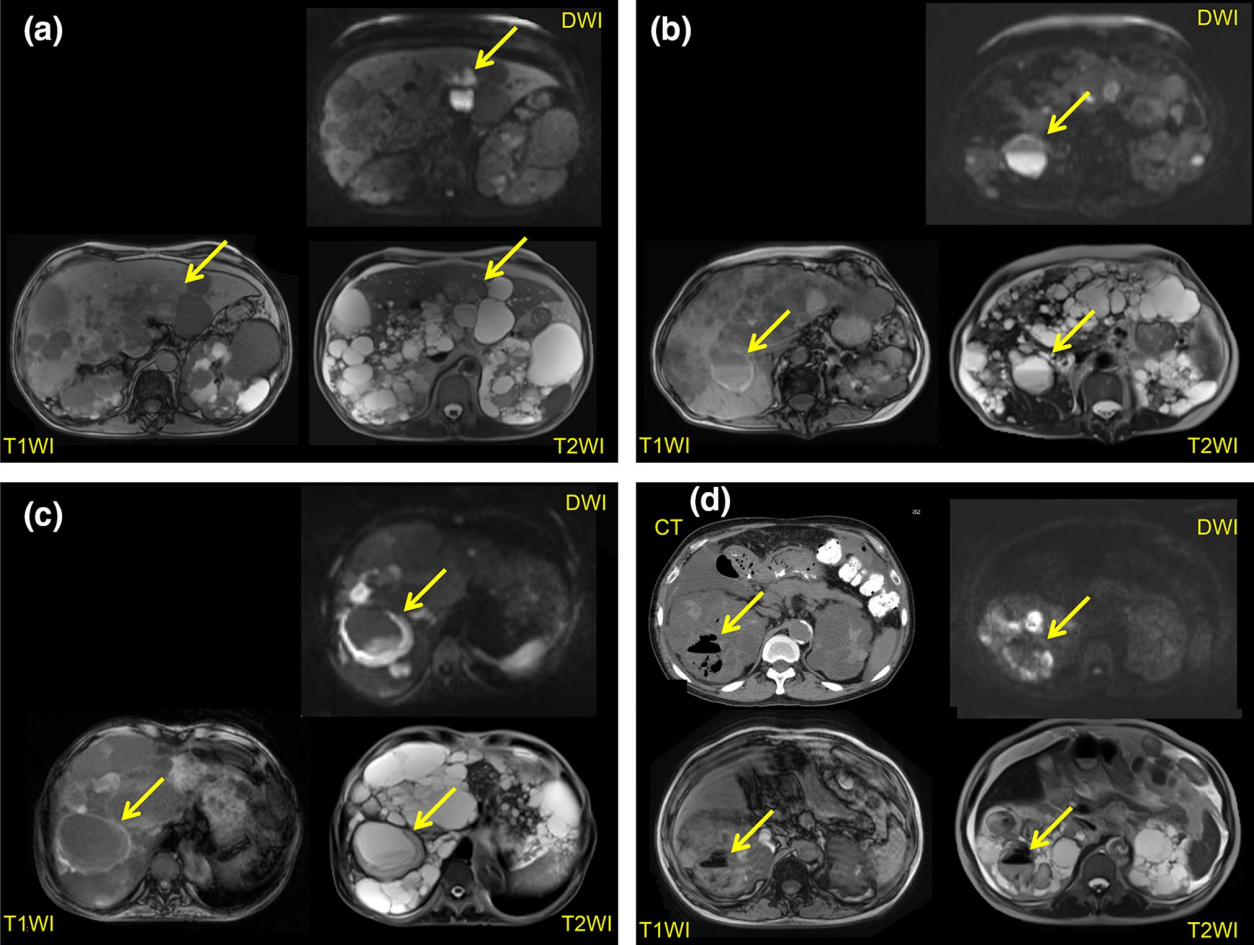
a MRI findings (T1WI, T2WI, and DWI) in a patient with cyst infection. The infected renal cyst shows a higher intensity on DWI compared with normal cysts, but it is difficult to identify on T1WI and T2WI. b MRI findings (T1WI, T2WI, and DWI) in a patient with cyst infection. A fluid-fluid level and cyst wall thickening can be seen. The infected renal cyst shows a higher intensity on DWI and T1WI than normal cysts, while it has a lower intensity on T2WI. c MRI findings (T1WI, T2WI, and DWI) in a patient with cyst infection. Obvious cyst wall thickening can be seen. The infected cyst is iso-intense on T1WI, T2WI, and DWI. d MRI findings (T1WI, T2WI, and DWI) in a patient with cyst infection. Gas is seen on T1WI, T2WI, and CT. The infected renal cyst shows a higher intensity on DWI compared with normal cysts, while it has a lower intensity on T2WI and T1WI. Sited from Suwabe T, et al. BMC Nephrol. 2016 Nov 9;17(1):170
